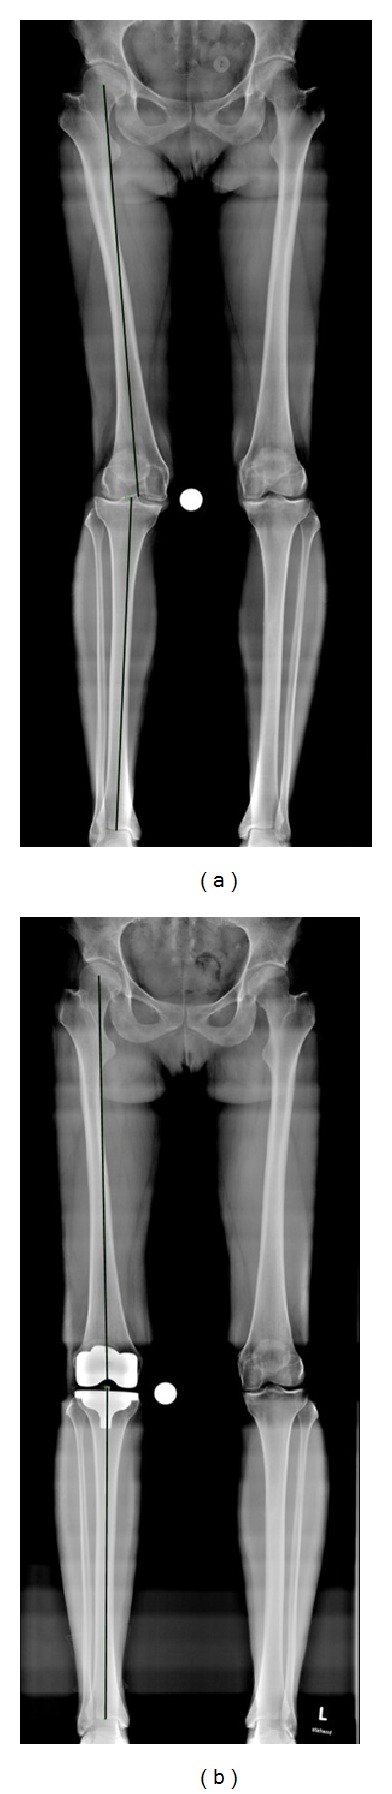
Mechanical leg axis before (a) and after (b) surgery on long-leg standing radiographs.
